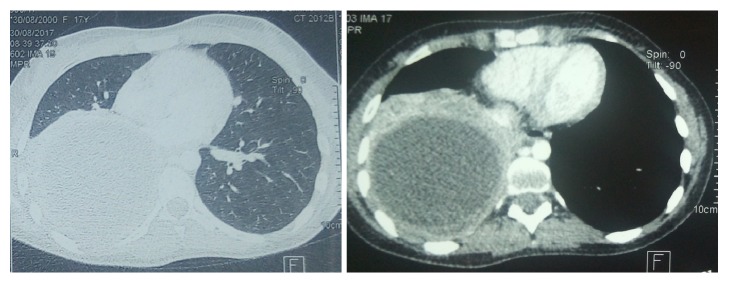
Thoracic CT scan showing a right basi-thoracic cystic image and a discreet slope with the pulmonary parenchyma with floating membrane aspect [right: parenchymal window, left: mediastinal window] (Patient 1).
